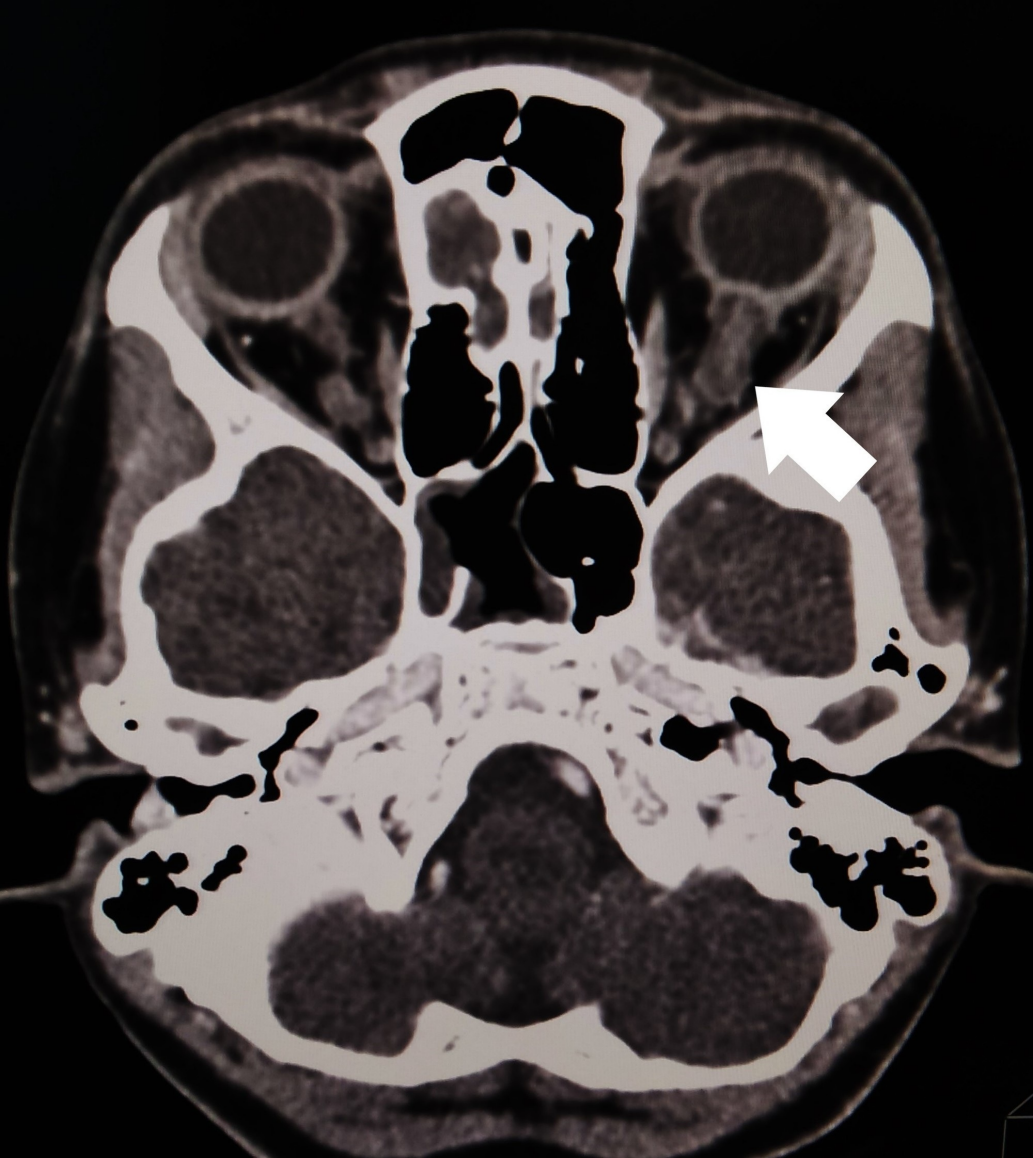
Computed tomography (CT) of the brain, orbit, and paranasal area Left optic nerve (arrow) is slightly prominent compared to the right side
